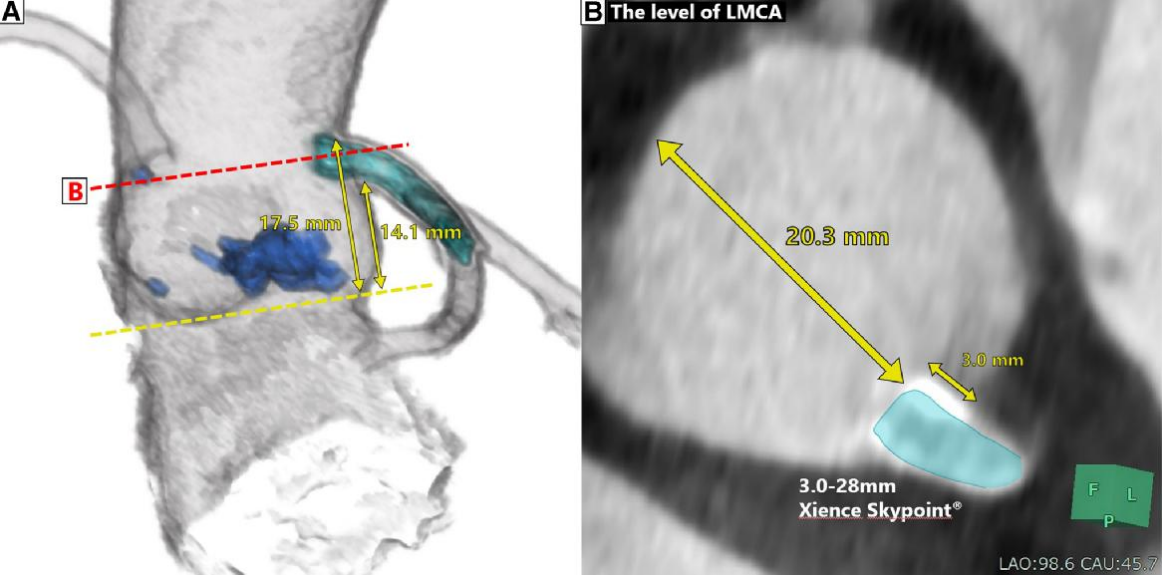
The preoperative computed tomography showed the height of the left main coronary artery from the annulus plane (*A*) and at the level of the left main coronary artery, there was a 3.0 mm protrusion of the left main coronary artery stent from the ostium with a distance of 20.3 mm to the opposite vessel wall was shown (*B*). CT, computed tomography; LMCA, left main coronary artery.
